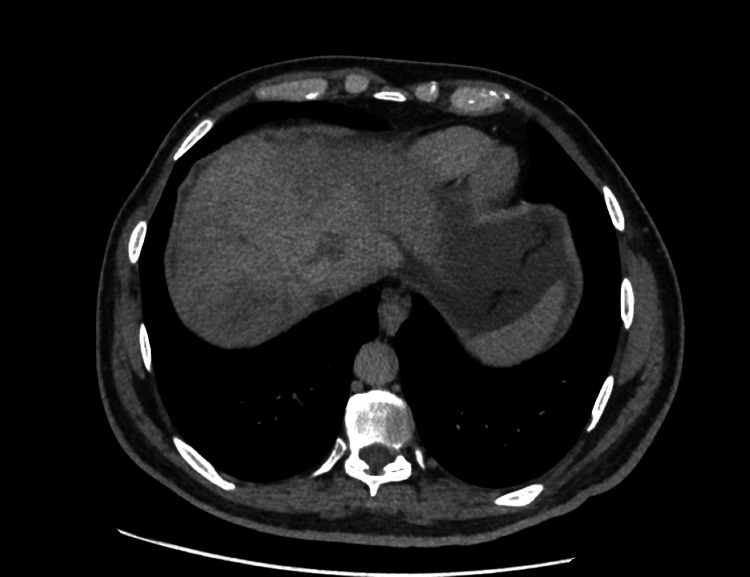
CT abdomen showed the general mild appearance of nutmeg liver that is characteristic of Budd Chiari syndrome (BCS)
